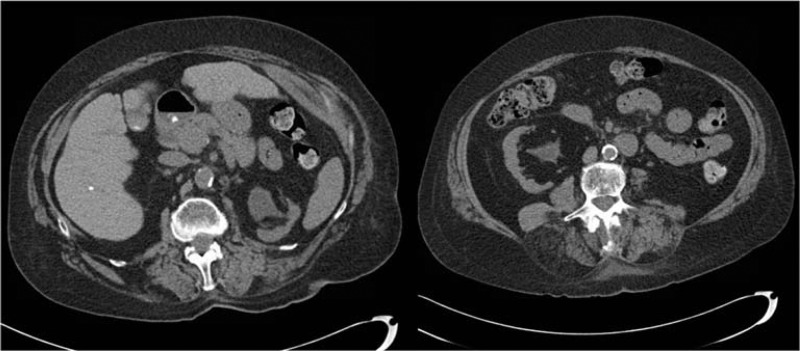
CT scan after 9 months of antibiotic therapy: No new left renal stones, improvement of left hydronephrosis and complete resolution of right renal stone.
